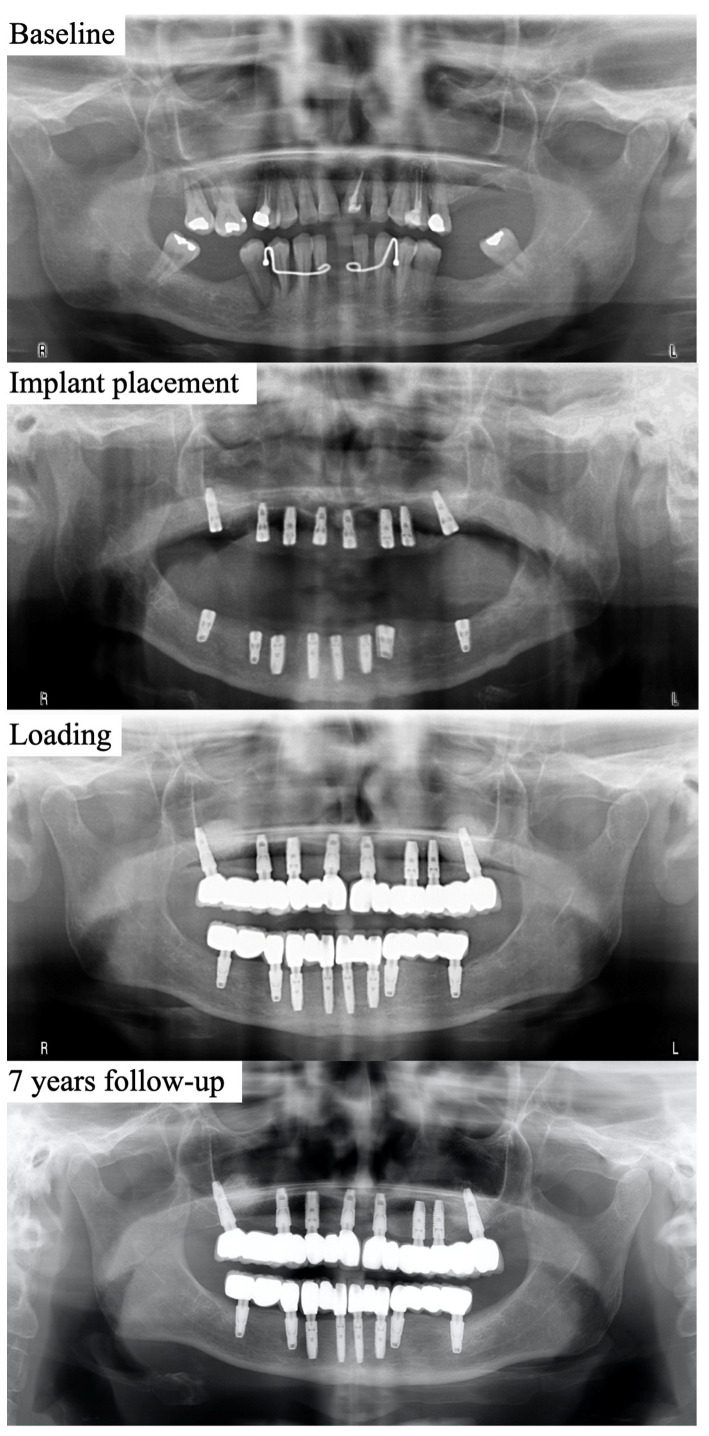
Representative series of radiographs from an included case.
